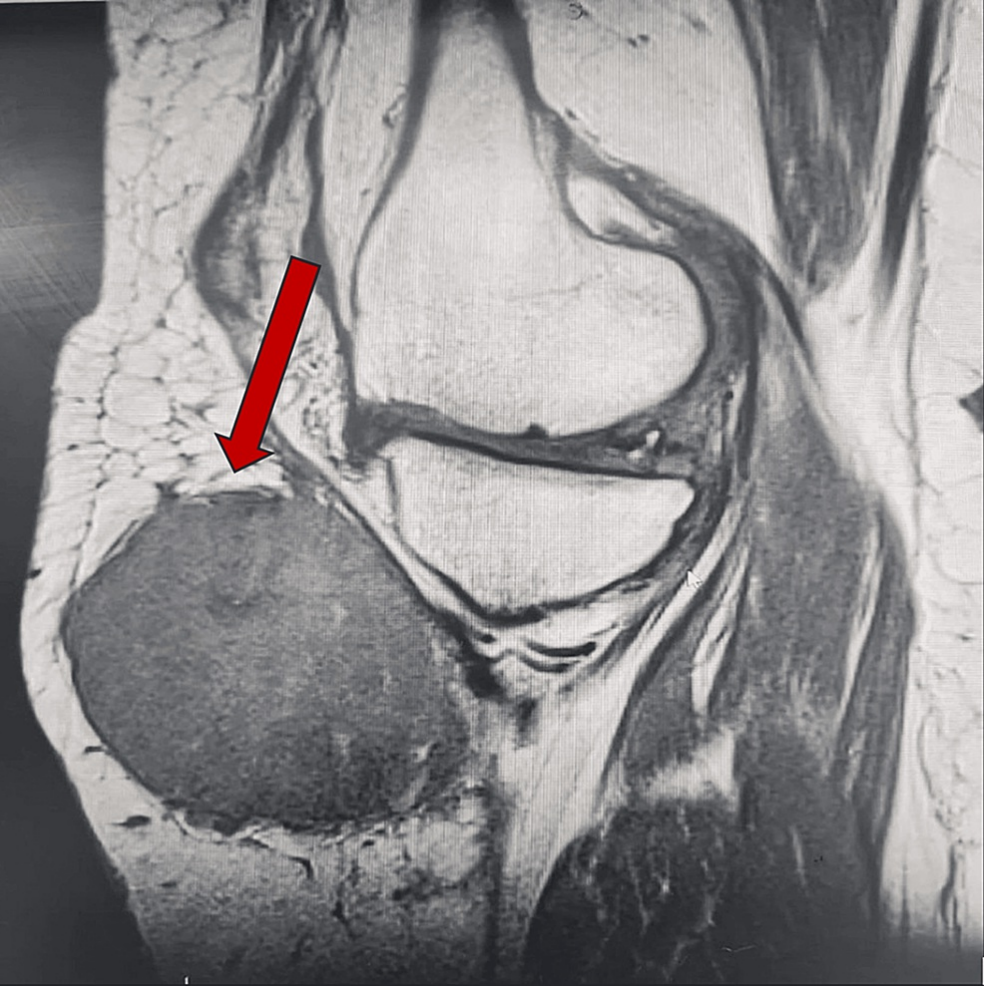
MRI Image showing a new mass on the anterior right thigh
